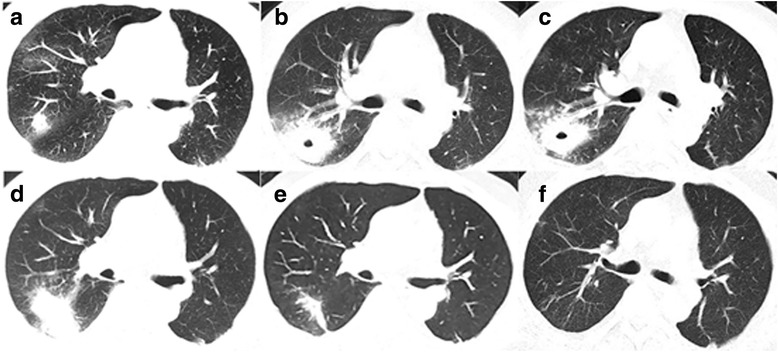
The patient’s pulmonary CT scan showed changes of the lesion during course of disease. a Right upper lobe nodule revealing recurrence of lung cancer confirmed by pathology (2016-3-21); b Right lung lesion became larger accompanied by an empty cavity after chemotherapy (2016-5-4); c No significant change of the right lung lesion after levofloxacin and cefepime treatment (2016-5-18); d No significant change of the right lung lesion after praziquantel treatment (2016-9-5); e Absorbed lesions after metronidazole treatment (2016-12-26); f No recurrence of amoeba during 6-month follow-up (2017-06-06)
